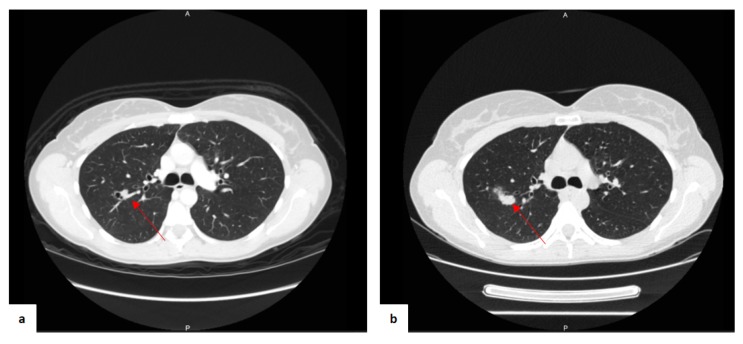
CT scan images of the first findings (a) and the findings after eight-month follow-up associated with antibiotic therapy (b). The nodule is indicated by the red arrow.
